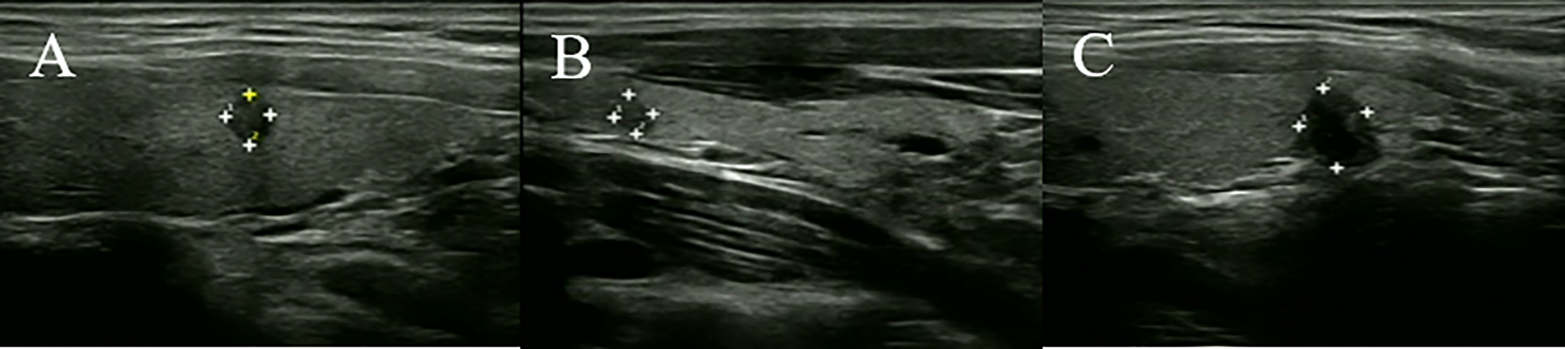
Ultrasound images of case X. (A) Nodule 1. (B) Nodule 2. (C) Nodule 3.
